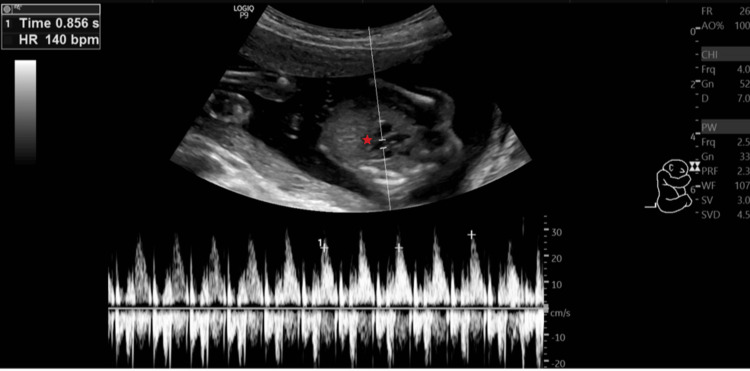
M-mode ultrasonography image demonstrating the fetal heart rate (~140 beats/minute). The star mark (*) shows the position of the fetal heart. The waveform of the fetal heart can be seen at the bottom of the image, and the fetal heart rate can be seen in the top left-hand corner of the image (140 beats/minute).
